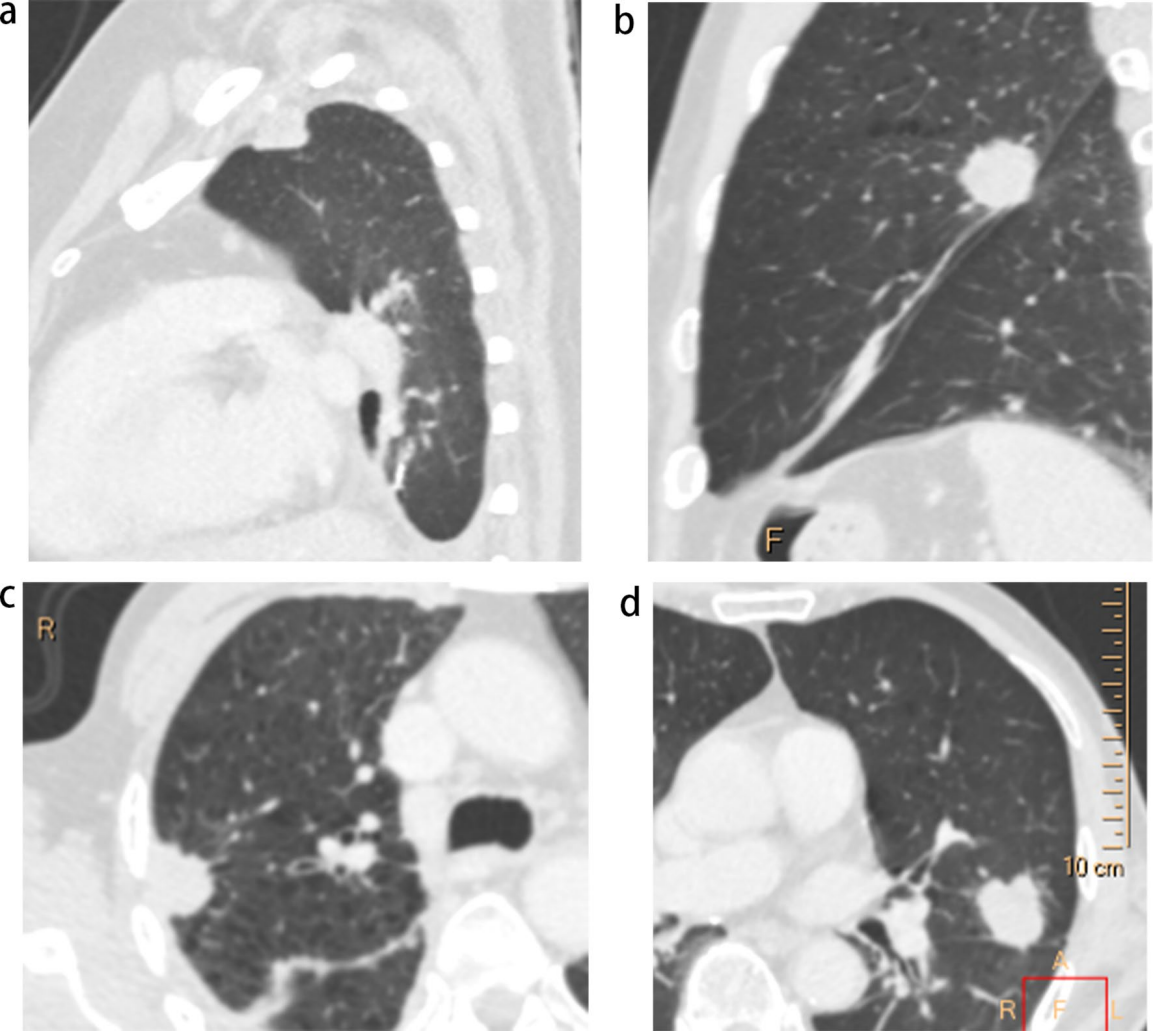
Representative CT images of the pleura being pushed and blunt angle. (a) Tumor straddled the costal pleura and partly occupied the extrapleural space. (b) Tumor pushed the interlobar pleura in the opposite direction. (c) Blunt angle between tumour and costal pleura (ventral side). (d) Sharp angle between tumour and interlobar pleura.
